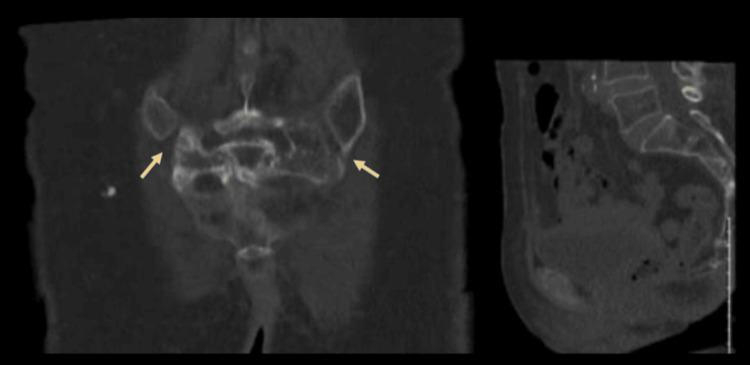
The pelvic CT scan reveals nondisplaced bilateral sacral fractures adjacent to the sacroiliac joints (yellow arrows), accompanied by a mild fracture of the anterior cortex of S2. Spondyloarthritic changes are also noted in the lumbar spine.
